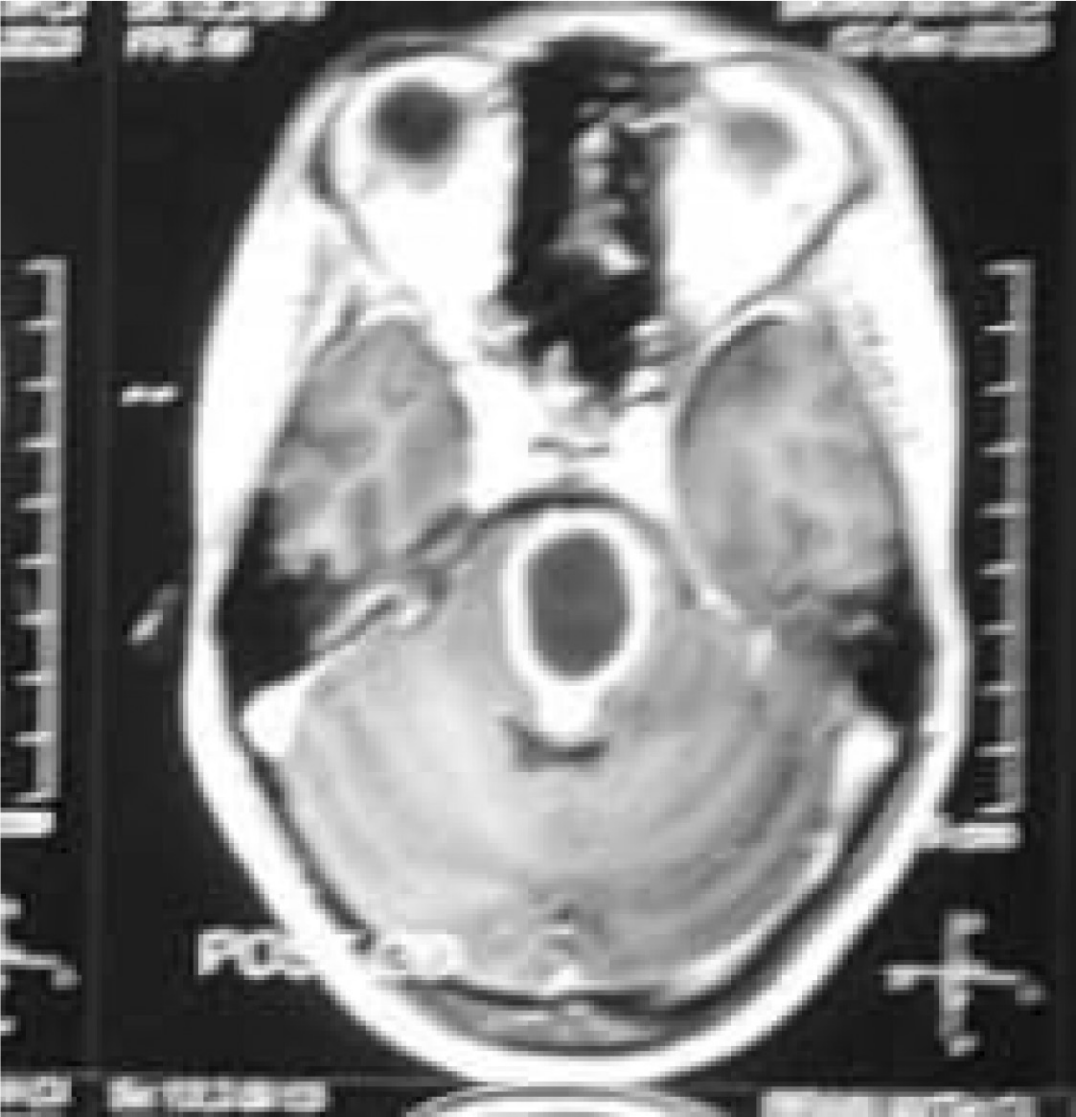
Contrast‐enhanced MRI in transverse section showing cystic lesion in the pontine area.
